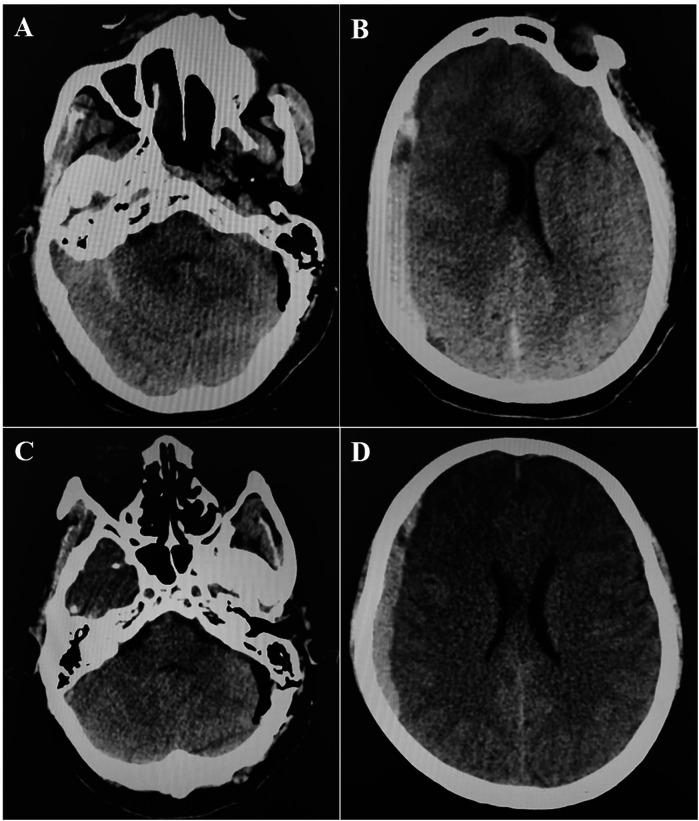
Postoperative CT scan. A,B: CT at 0 h post-operation showed that there was no hemorrhage in the operation area of left cerebellopontine angle, but there was right supratentorial subdural hematoma (1 cm thickness). C,D: CT at 4 h post-operation showed that hematoma was stable.
